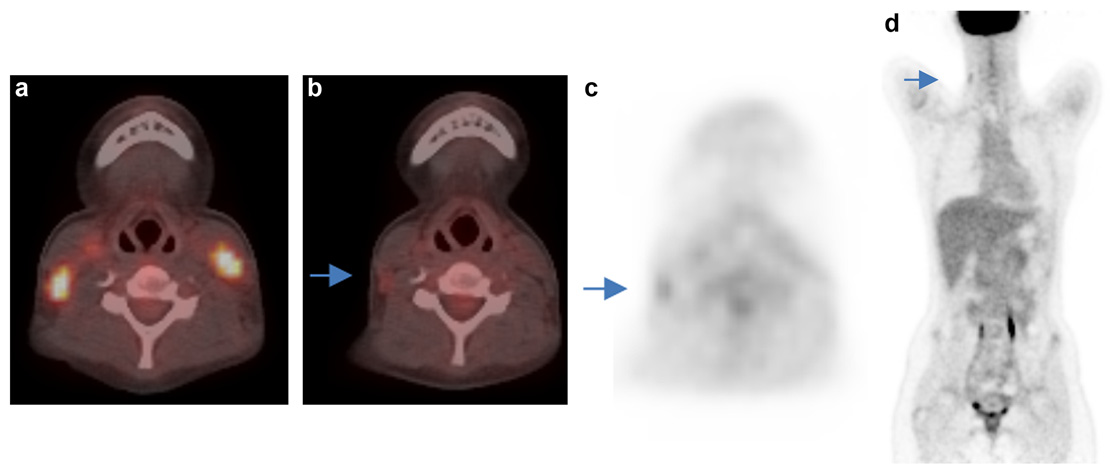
Discrepant D scoring of the neck region. Case 44: a) Lymphoma involvement at both sides of the neck before treatment. b) and c) residual lymph node on the right neck with slightly enhanced FDG uptake after to cycles ChT. d) The residual uptake is less than in the liver, but it is difficult to decide if it is above or below the MBP uptake. Deauville score of the readers R1-5, respectively: 2-3-3-1-3. Courtesy Dept. Clinical Physiology, Sahlgrenska University Hospital, Gothenburg Sweden.
